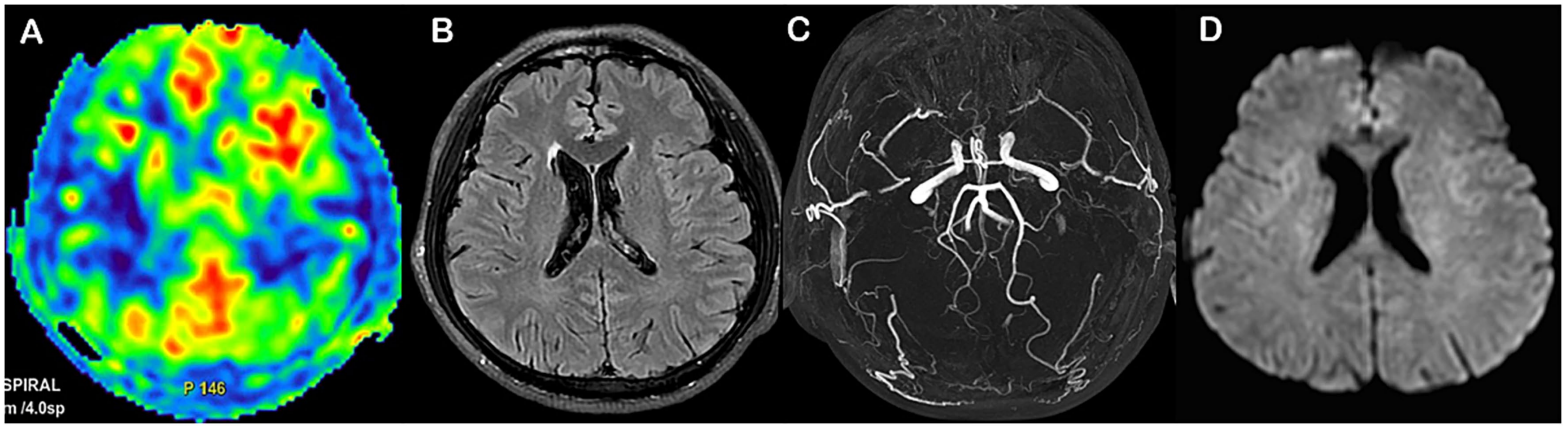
Representative MMD case with good prognosis (A–D). Male, 50 years old, with an admission mRS score of 1, and the 1-year mRS score was 0. FLAIR image showed no ivy sign (A); A Preoperative ASPECTS score was 7 (B); Postoperative Houkin score was 3 at 1 week, and MRA staging was stage 2 (C); Follow-up DWI showed no high signal (D). The nomogram score was 25, with a probability of poor prognosis < 0.1.
